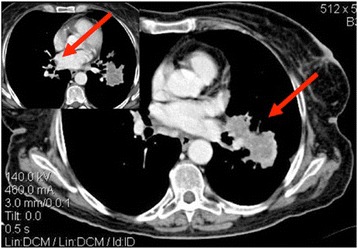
Baseline thorax-abdomen-pelvis computed tomography, showing a large mass in the left lower lobe, multiple bilateral pulmonary nodules and mediastinal adenophaties.
